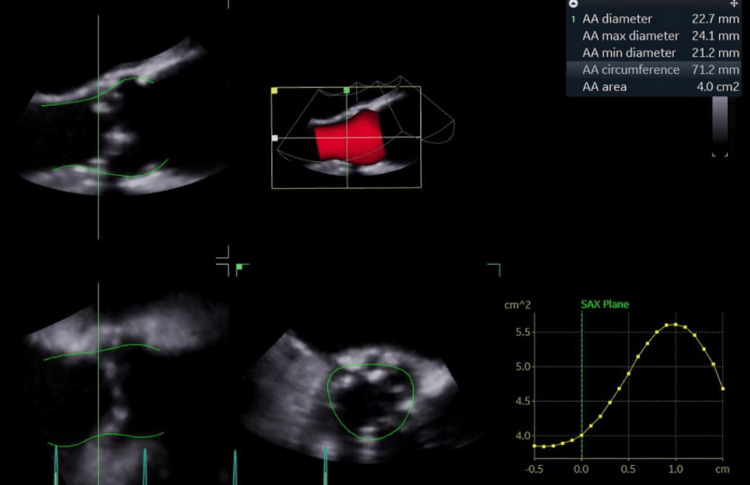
Transesophageal echocardiogram TAVR Sizing TAVR: Transcatheter aortic valve replacement
